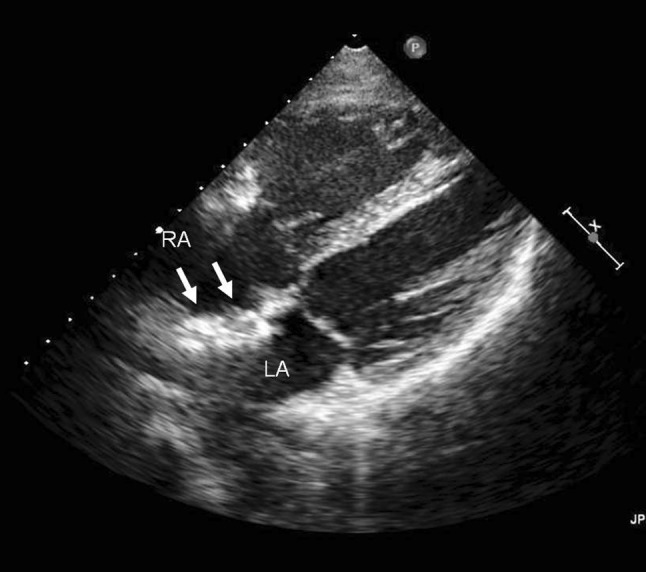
Echocardiogram at the onset of migraine. The device (*arrows*) was positioned in the atrial septum with no evidence of thrombus. *RA* right atrium, *LA* left atrium
